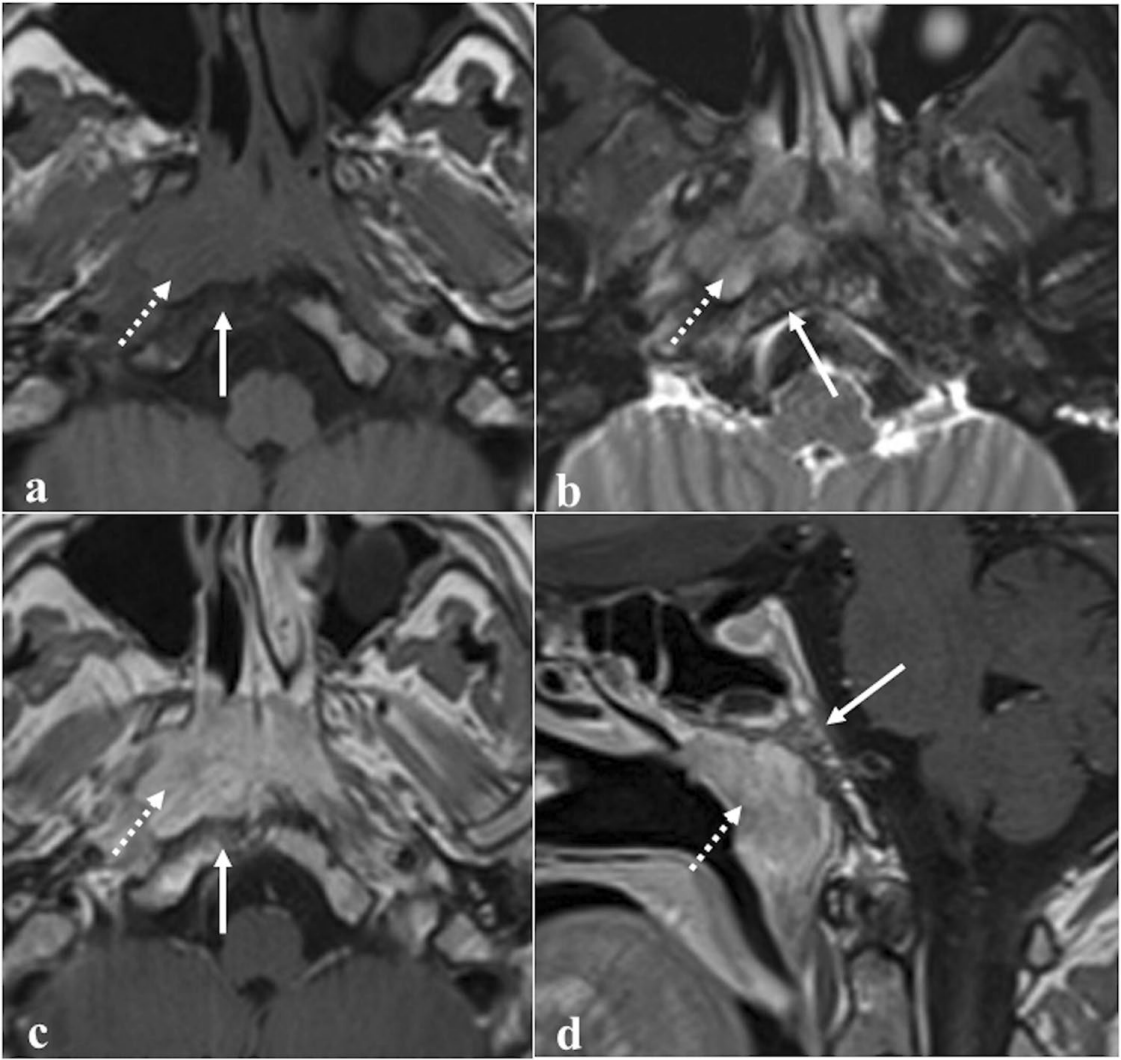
Nasopharyngeal carcinoma in a 28-year-old male patient. MRI shows a lesion of the right Rosenmüller fossa (white dotted arrows) invading the clivus posteriorly. Bony invasion (white arrows) is better depicted on axial T1W non-CE image as a focal area of low SI in the clivus next to the primary tumour (a). Bony involvement is less noticeable on axial T2W (b), axial (c), and sagittal (d) T1W CE images
